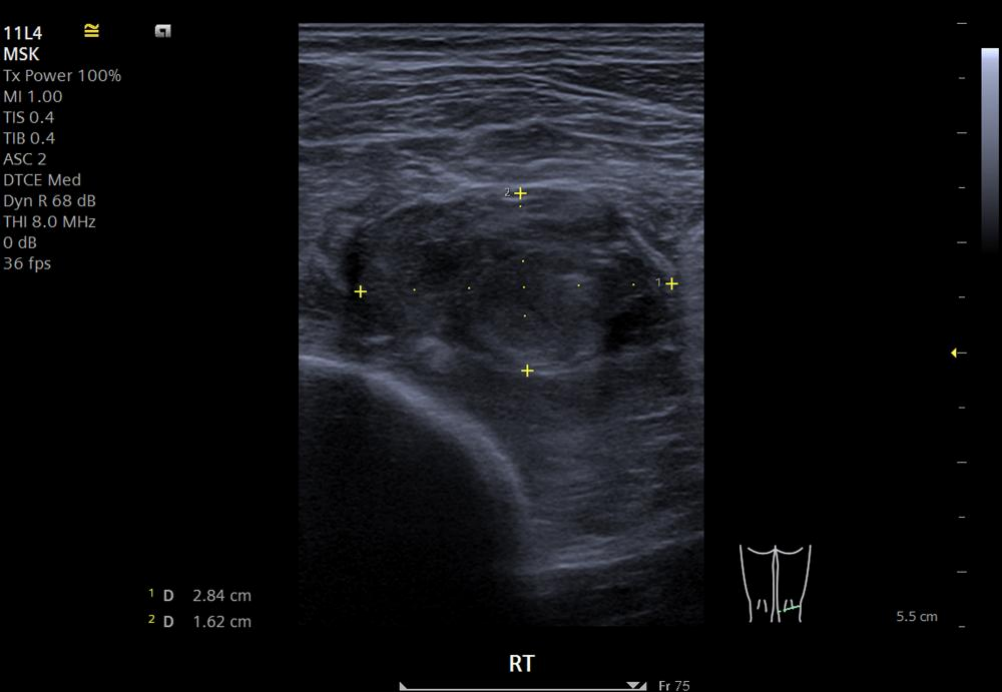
Ultrasound-guided drainage of right soleus muscle abscess.
